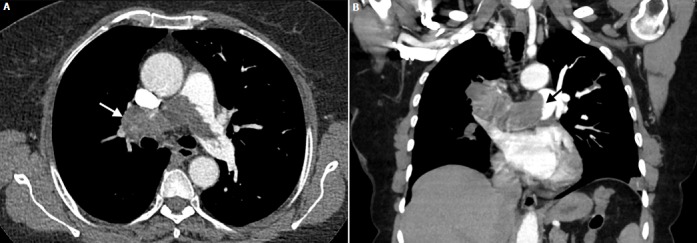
Axial (A) and coronal (B) CT angiography images: A) a large low density filling defect occupying lumen of the pulmonary trunk, right and left pulmonary arteries with expansion of right pulmonary artery (white arrow); B) protrusion of the proximal end of the lesion is also observed (black arrow)
